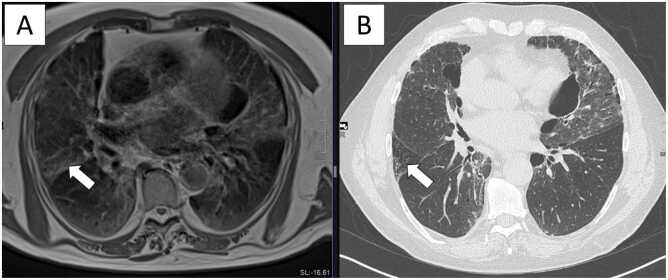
Clinical Case #1: 72-year-old male patient with rheumatoid arthritis-related interstitial lung disease. The depicted scoring level is level 3 (pulmonary venous confluence). The patient underwent (A) low-field MRI of the lung with a proton density weighted sequence and (B) HRCT of the chest on the same day. Overall disease extent was estimated almost equal on MRI and HRCT (MRI, median 48 %; HRCT, median 49 %) and included areas of honeycombing and ground-glass opacities as predominant patterns in this particular patient. The extent of ground-glass opacity was slightly overestimated (MRI, median 41.1 %; HRCT, median 36.5 %), and reticulation was underestimated on MRI compared to HRCT (MRI, median 8.1 %; HRCT, median 10.5 %). Particularly in the right lung, areas of reticulation visible on HRCT rather appear as ground-glass opacity on MRI (arrows).
